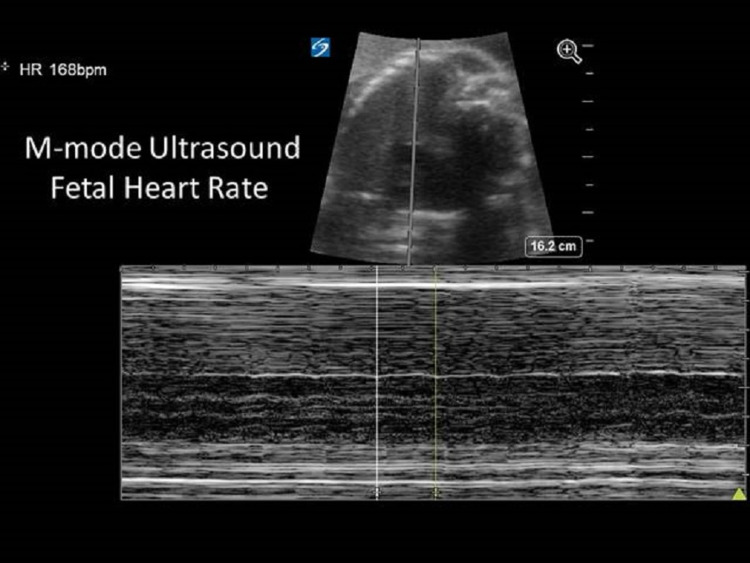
Representative M-mode ultrasound showing fetal heart rate.
